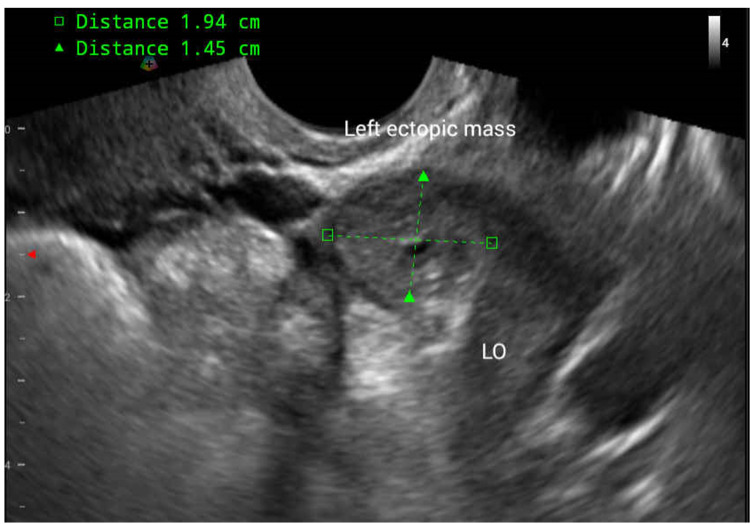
TVUS at the emergency department. Left tubal ectopic mass measuring 1.9 x 1.5 cm. Compared with the previous TVUS images, the size of the mass had shrunk after three doses of MTX. TVUS: transvaginal ultrasonography; MTX: methotrexate.
